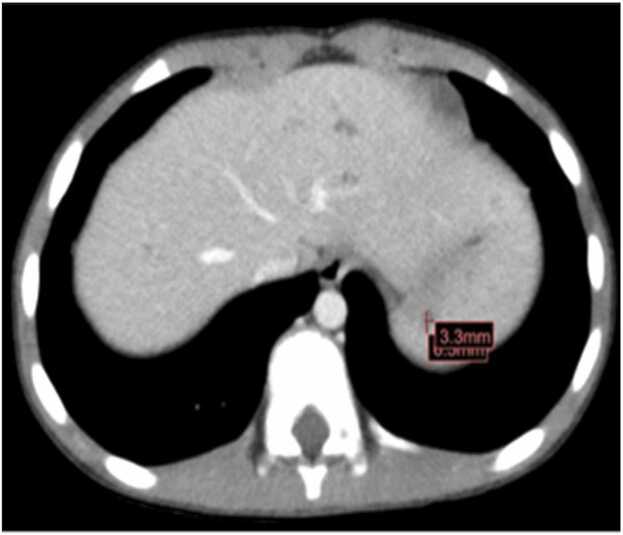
Case 6. CT abdomen with innumerable small hypoattenuating foci throughout the spleen.
